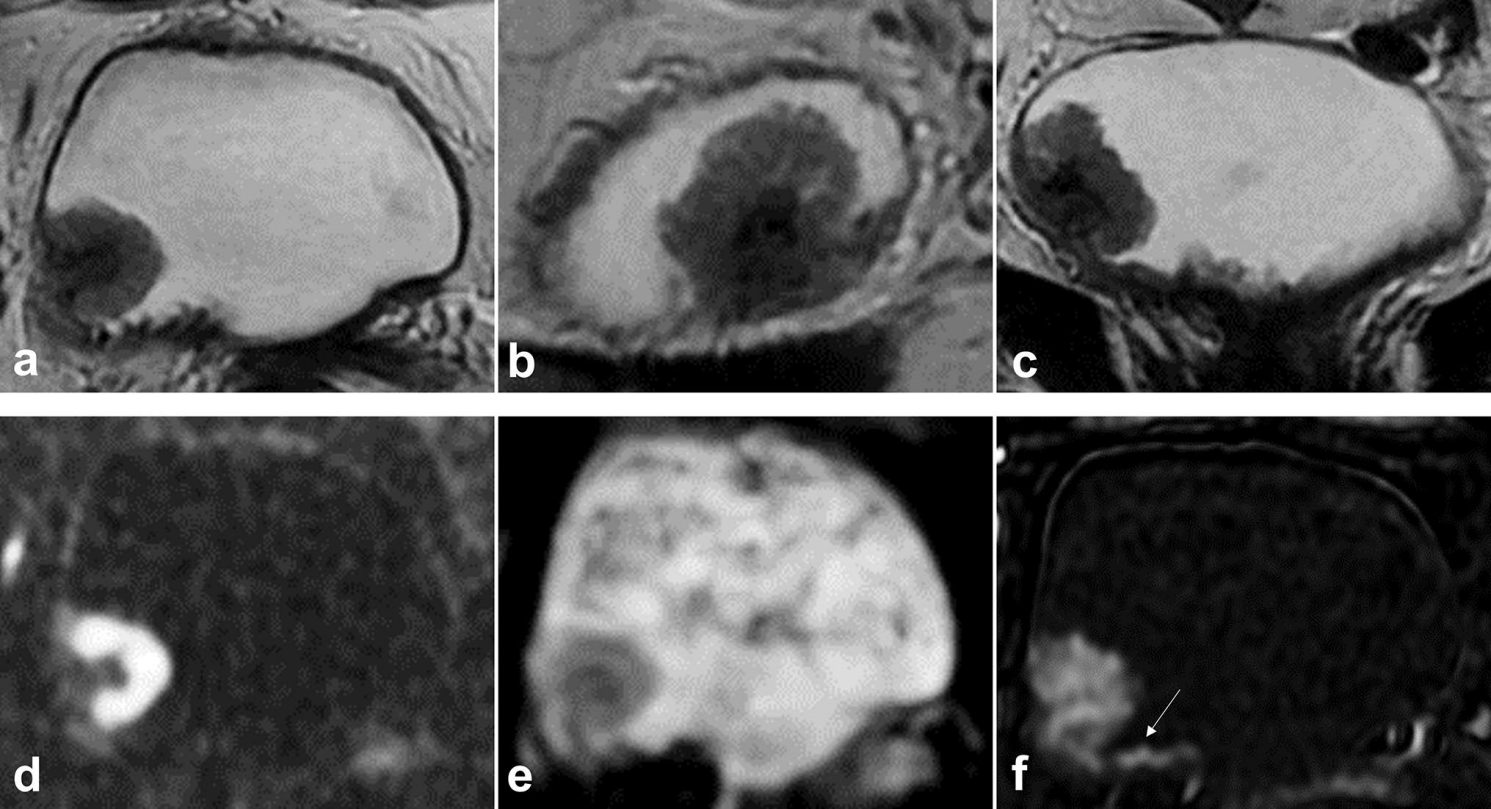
Case example of a 66-years-old female that was incorrectly scored as a VI-RADS 4 by the less experienced readers, but correctly scored as VI-RADS 2 by the more experienced readers. (a–b–c) Axial, sagittal and coronal T2-weighted imaging showing a pedunculated bladder tumor (20 mm) at the right lateral wall; (d–e) diffusion-weighted imaging and ADC map showing the “inchworm sign”, typical of non-muscle-invasive bladder cancer; (f) dynamic contrast-enhanced MRI showing integrity of the *muscularis propria* layer and enhancement of the “inner layer” (arrow). The lesion should be classified as VI-RADS 2. VI-RADS, Vesical Imaging-Reporting and Data System; ADC, apparent diffusion coefficient
